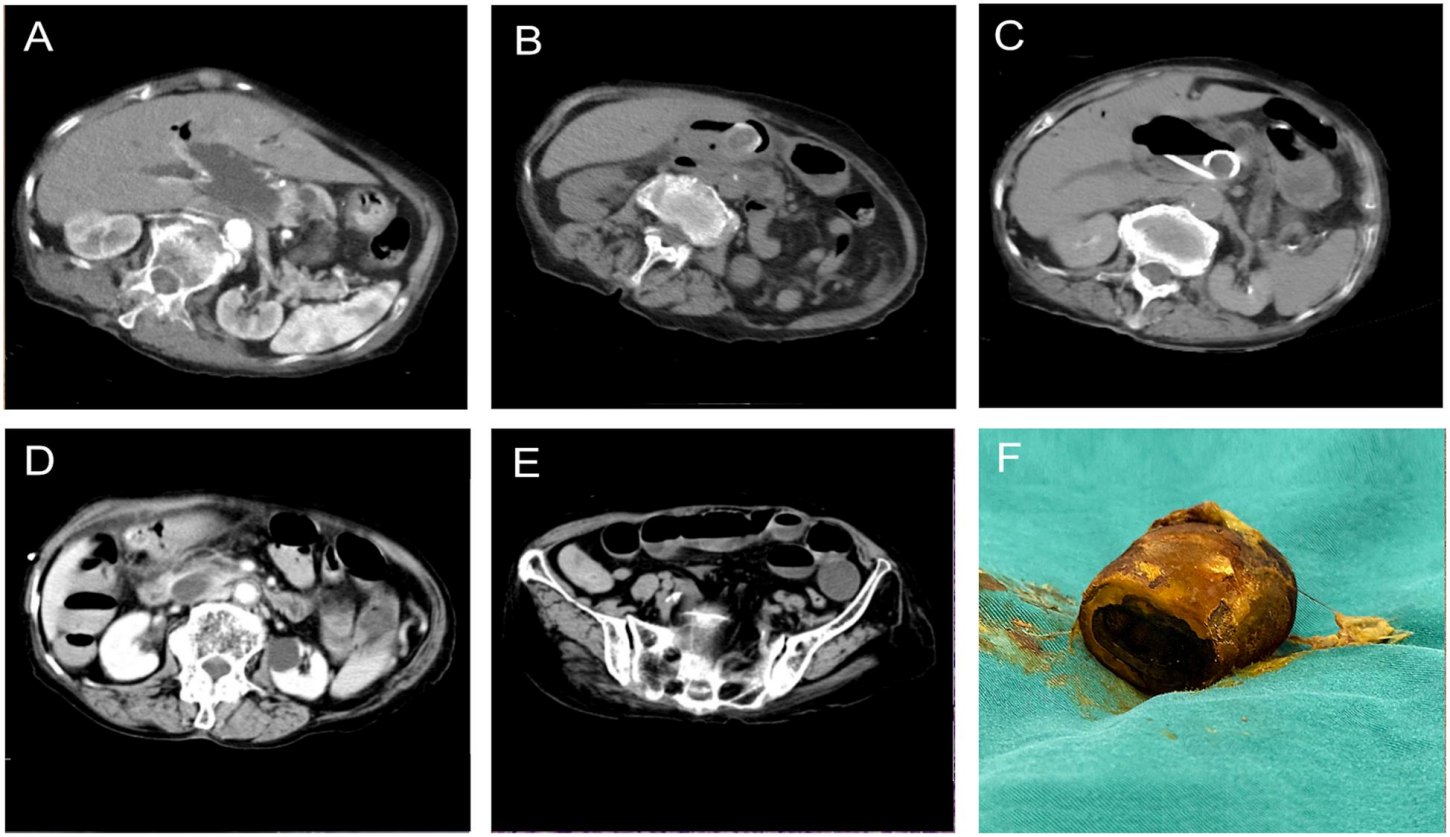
Computed tomography revealed dilation of the common bile duct with pneumobilia (A). Computed tomography revealed a suspected cholecystogastric fistula with an ectopic gallstone located in the gastric antrum (B). Computed tomography revealed the percutaneous transhepatic cholangial drainage was placed in the dilated common bile duct (C). Re-evaluation computed tomography revealed the gallstone in the gastric lumen had disappeared (D). Computed tomography revealed an impacted radiopaque gallstone accompanied by its proximal bowel loop dilation, consistent with gallstone ileus (E). The surgically removed gallstone was hard in texture, oval in shape, and yellowish-brown in color, measuring 3.3 cm in maximum diameter (F).
